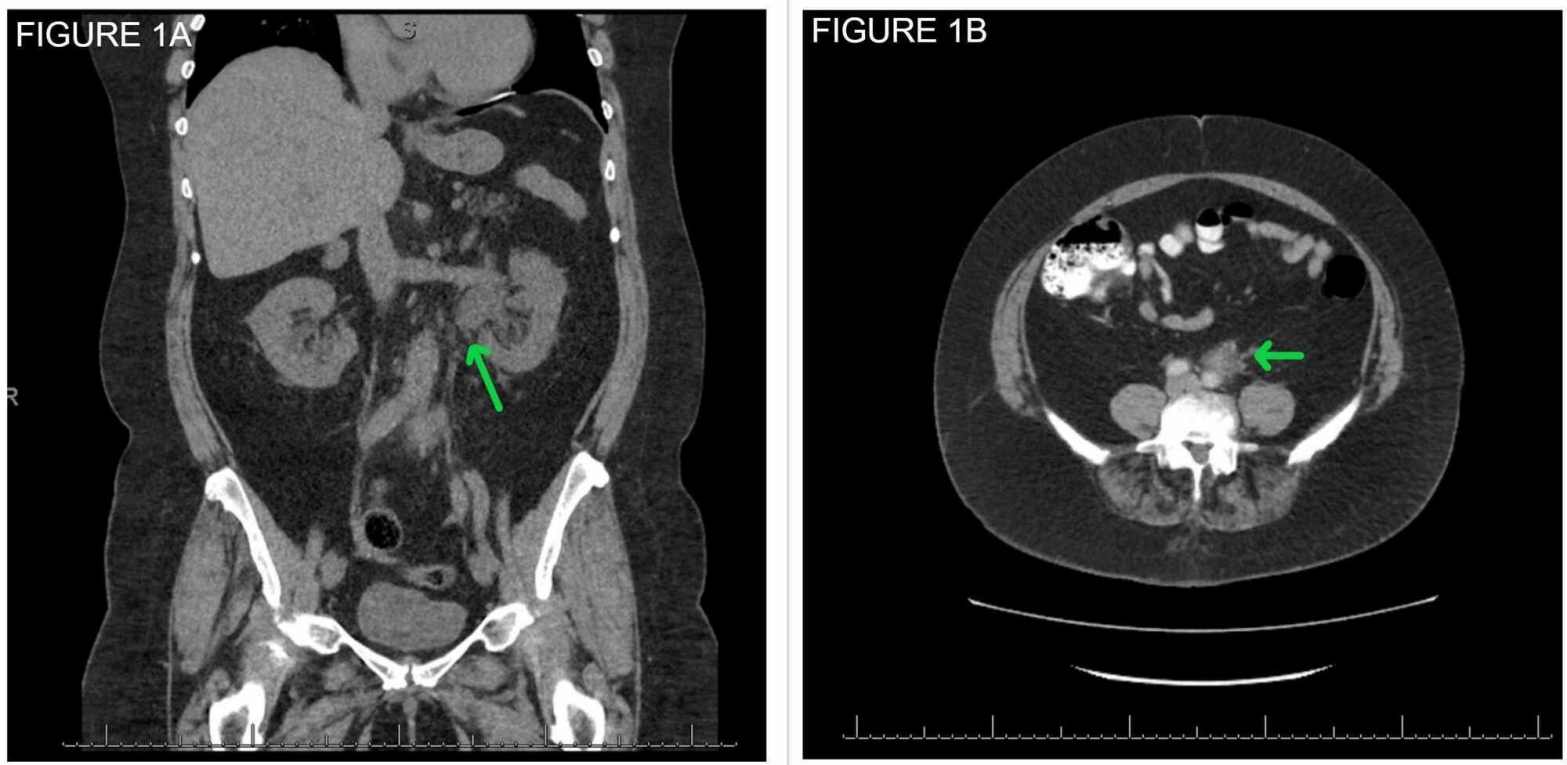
Initial CT scan of the abdomen and pelvis showing (A) Hydronephrosis on the left due to a retroperitoneal mass. Note the left-sided hydronephrosis and proximal hydroureter and (B) A 2.8 x 1.7 cm retroperitoneal soft tissue mass along the anterior margin of the aorta and abutting the mid-segment of the left ureter
